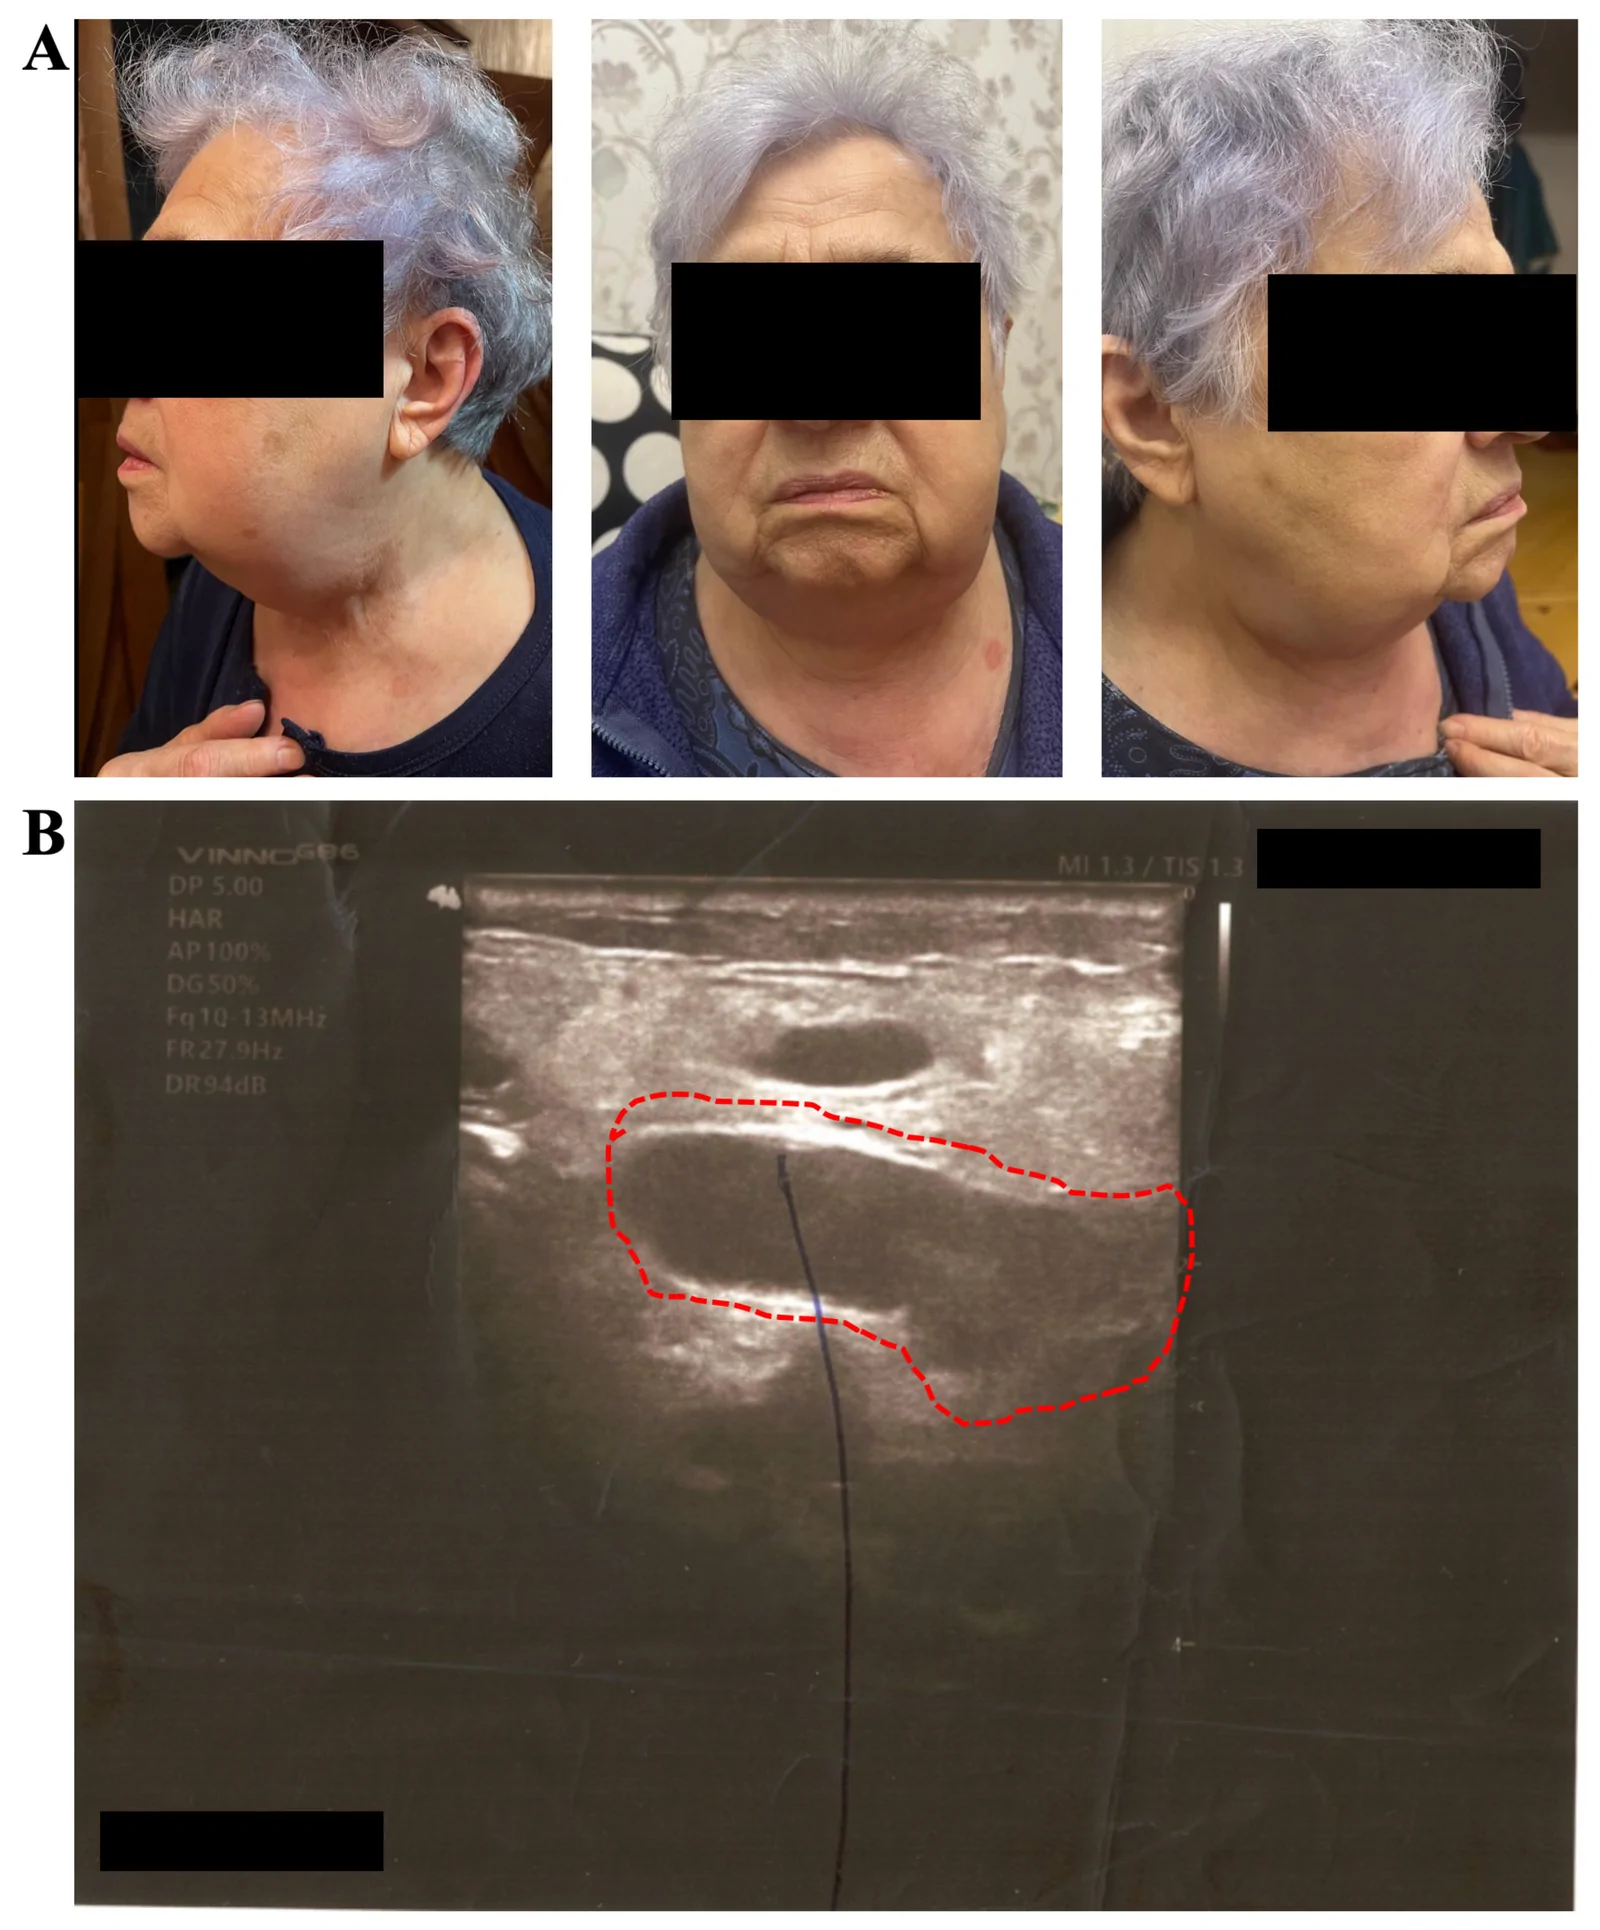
(A) Clinical presentation of the patient on the morning of the first day of symptom onset, showing facial asymmetry due to swelling on the left side of the face. (B) Ultrasound image of the left submandibular salivary gland on day 6 after symptom onset, demonstrating marked ductal dilatation up to 0.8 cm (approximately 13.33 times larger than the right submandibular duct), highlighted by the red dotted line.
